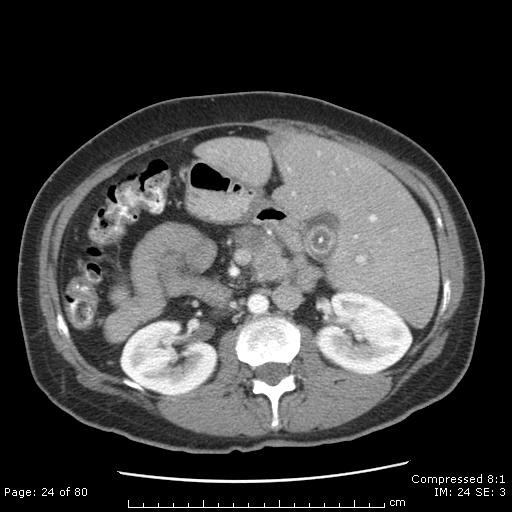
Diagnostic CT scan. CT scan obtained 8 months later when the patient presented with jaundice and a bulging Ampulla of Vater on ERCP. A new 4.2 cm mass is now present in the pancreatic head, obstructing the common bile duct.
